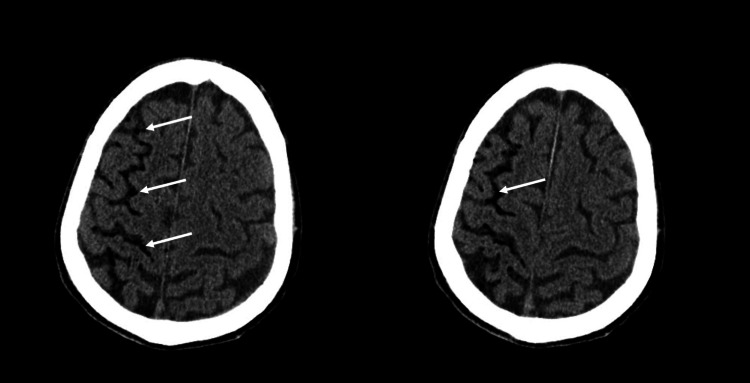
Cerebral CT performed prior to hyperbaric treatment. The white arrows highlight the air emboli.
